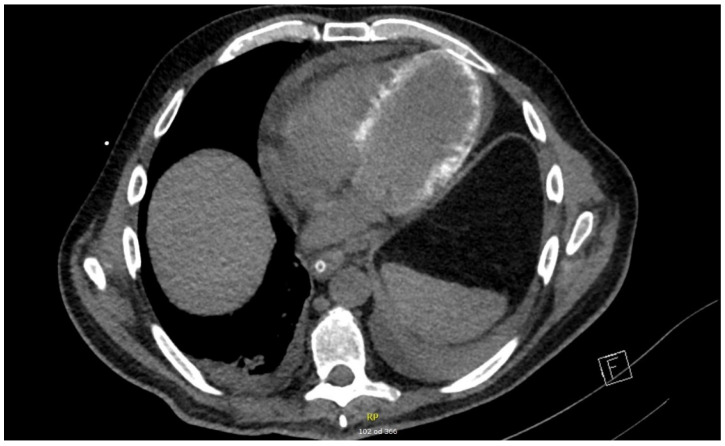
Cardiac CT scan with diffuse myocardial calcinations and pericardial effusion, performed on day 20 of ICU treatment (axial image).
